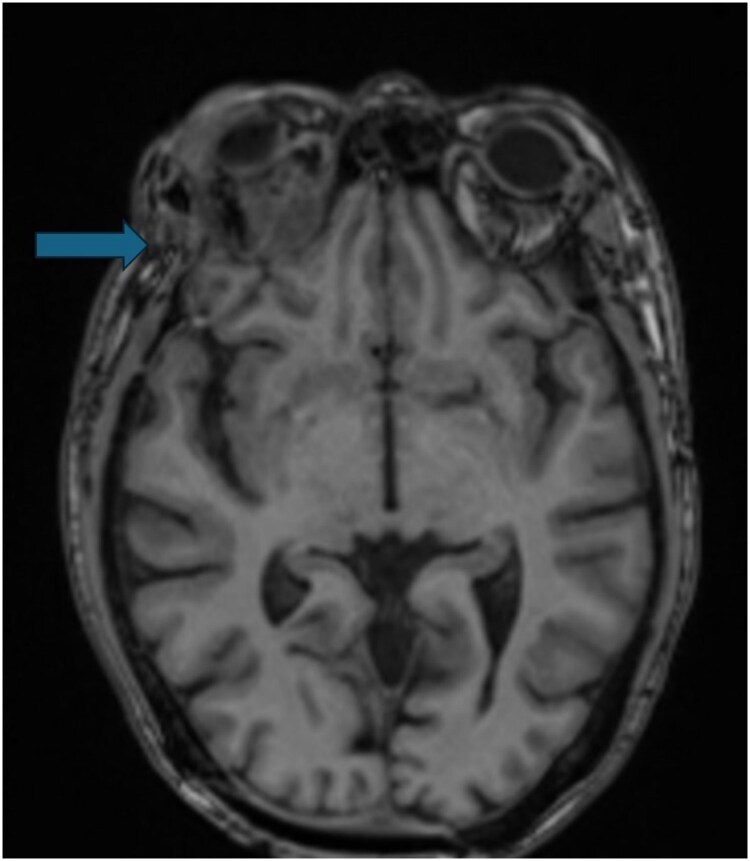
Magnetic resonance imaging of the brain showing a mass-like enhancement along the right orbital cavity with thickening/enhancement of the occipital dura and an adjacent enlarging 0.7-cm enhancing occipital calvarial metastatic lesion.
